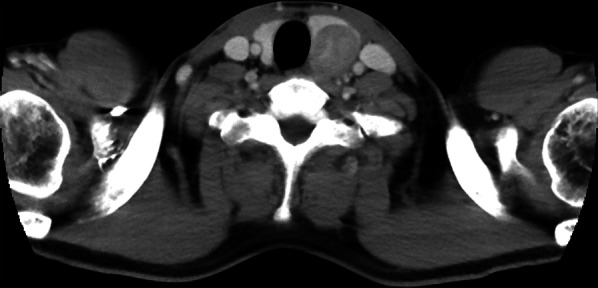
Enhanced CT of the neck. The mass lesion shows poor contrast on the posterior side of the left lobe of the thyroid. No extraglandular infiltration or cervical lymphadenopathy is observed
